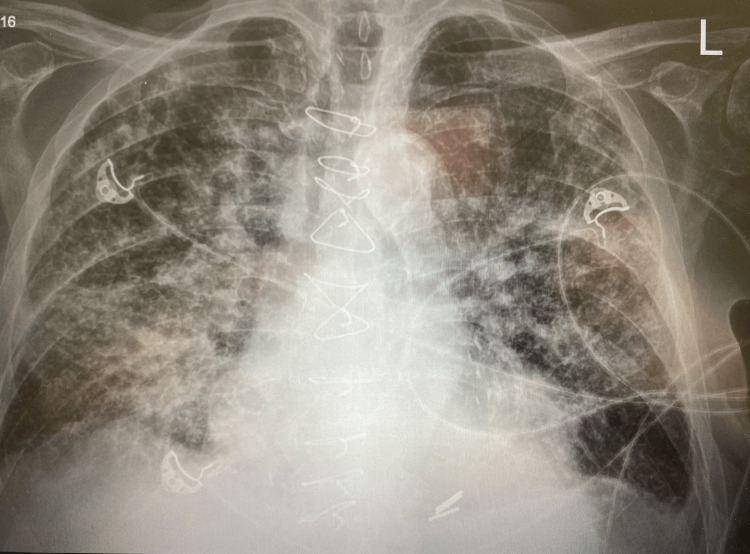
Chest X-ray showed new multifocal consolidations with interstitial markings and alveolar edema throughout both lung fields.
